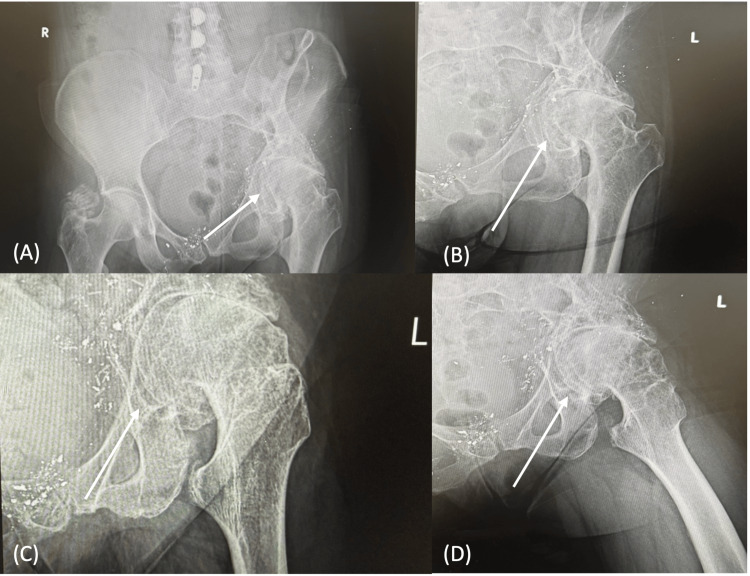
Preoperative hip and pelvis radiographs. (A) Anteroposterior (AP) pelvis radiograph demonstrating severe end-stage left hip osteoarthritis with complete joint space loss, pelvic obliquity, femoral head deformity, and retained metallic fragments from a prior gunshot wound.
